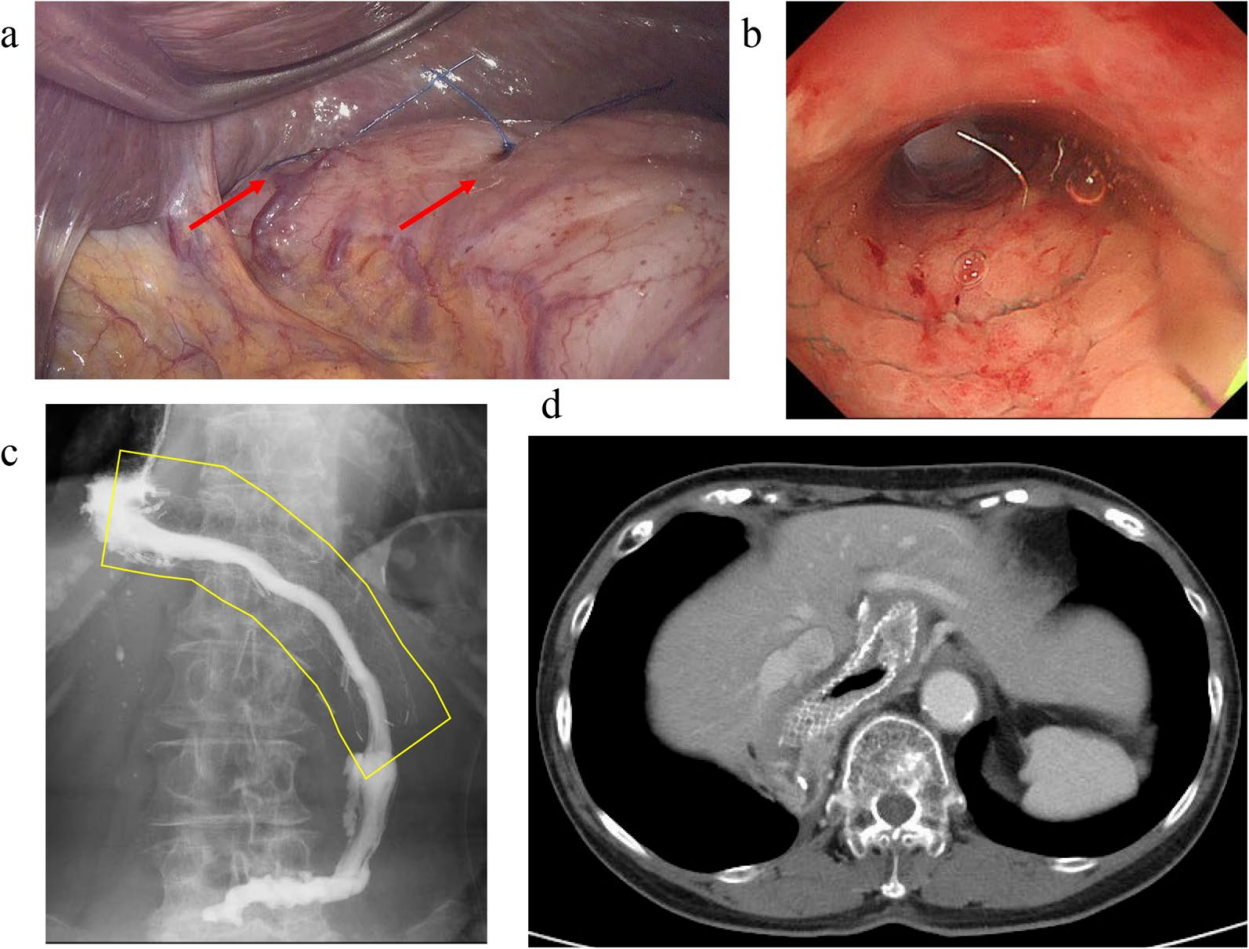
Findings during laparoscopic stent fixation (LSF). a We fixed the stent through the full-thickness gastric wall with 3-0 Prolene at the oral side of the antrum and lower gastric body (red arrows). b Needle placement through the stomach wall was confirmed by endoscopy. c The stent (area outlined in yellow) was patent after surgery, and contrast medium passed through easily. d Postoperative CT showing no dilated gastric tube in the thoracic cavity. *CT* computed tomography
